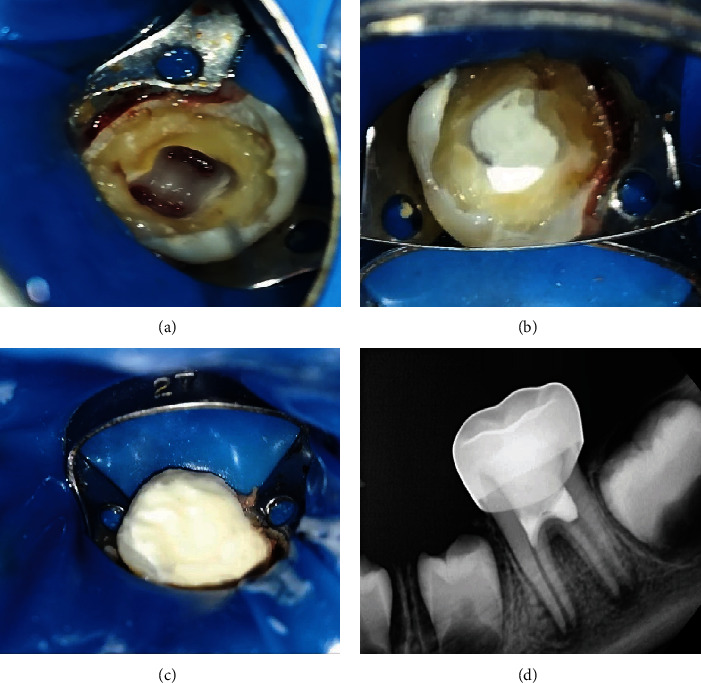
Pulpotomy procedures: (a) hemostasis achieved, (b) bioceramic putty application, (c) building coronal part with GIC, and (d) postoperative periapical radiograph.
